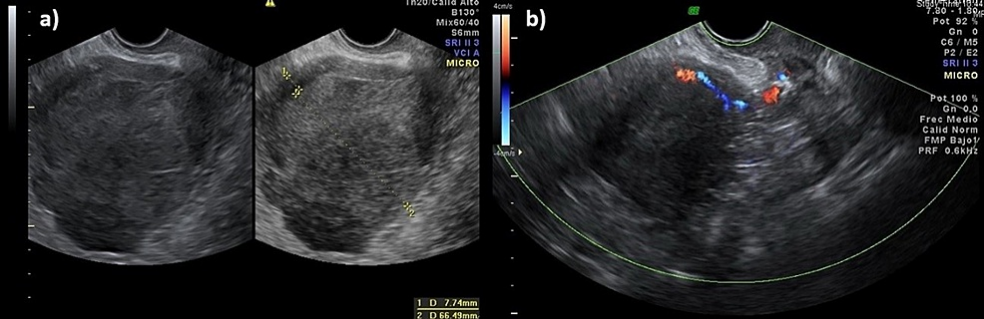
Transvaginal Doppler ultrasound A) Anteverted uterus enlarged with hysterometry of 107x73x90 mm, heterogeneous myometrial walls at the expense of subserous myoma at the level of the uterine fundus measuring 38x22x37 mm. 66mm thickened endometrium. A 3 mm thinning of the posterior myometrial face is observed with loss of the endometrial-myometrial interface. Endometrial/myometrial relationship 33/47 of the posterior face. The anterior myometrial thickness of 10 mm, showing the endometrial-myometrial interface. B) Endometrium with type two Doppler score on the anterior side.
